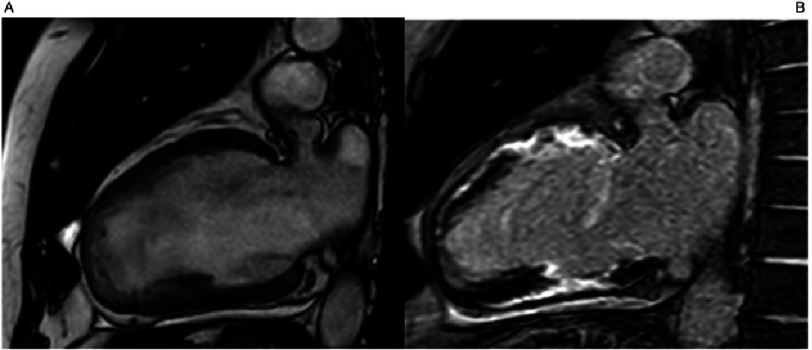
Cardiac Magnetic Resonance (CMR) Apical 2CH view: A) Cine and B) Late gadolinium enhancement images. End-stage HCM with progressive remodelling and sever systolic dysfunction, atrial fibrillation and ventricular tachycardia. MYH7 p.Arg453Cys.
